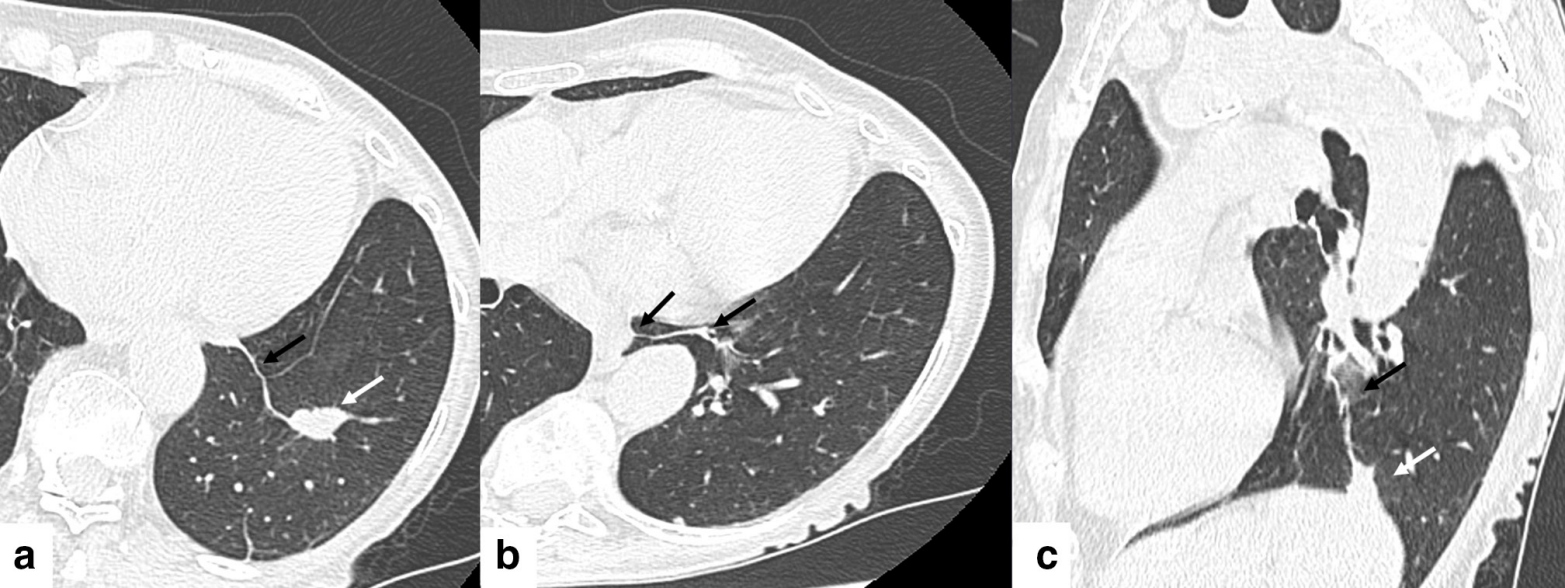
A-C. 75-year-old asymptomatic female with biopsy-proven granulomatous lymphadenitis. 3A: Axial chest CT image on lung windows showing a 2 cm lobulated solid nodule (white arrow) within a septal extension of the left IPL (black arrow). 3B: Axial chest CT image on lung windows at a higher level showing medial extension of the IPL along the left side of the esophagus (black arrows). 3C: Sagital oblique chest CT image on lung windows showing a 2 cm nodule (white arrow) at the base of the IPL, and superior extension of the IPL (black arrow) towards the lower hilar pole.
